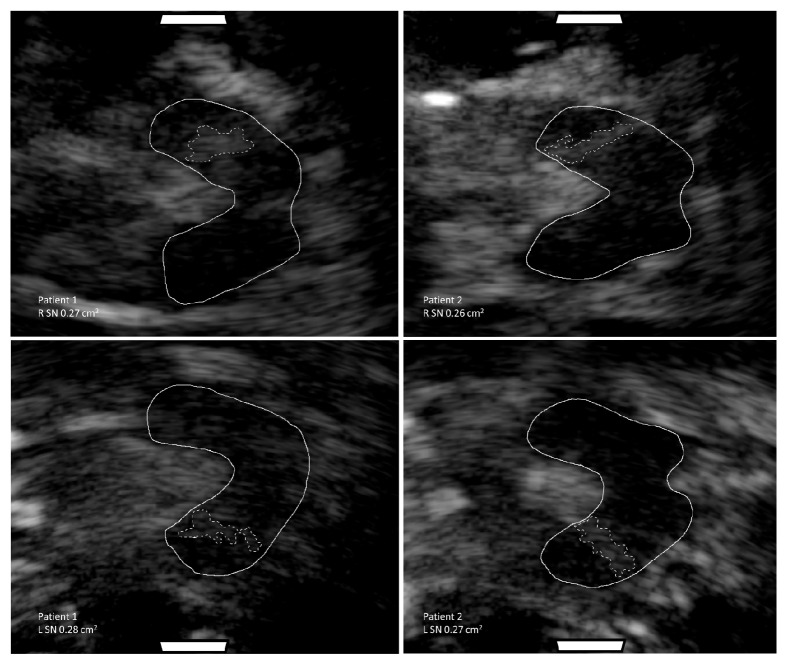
Transverse view of substantia nigra (SN) morphology in Patients 1 and 2 using transcranial sonography. Images of the right (top) and left (bottom) SN are shown. Solid line encircles the mesencephalic brainstem. Dotted line encircles the SN. White rectangles correspond to the placement of the probe.
